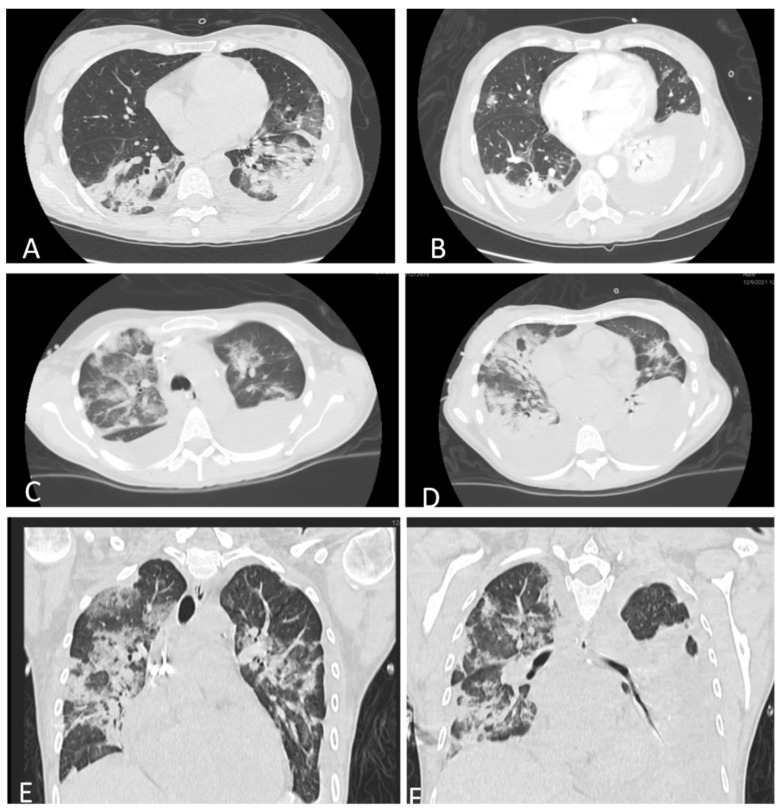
Thoracic CT scan. (A) Native thoracic scan showing bilateral COVID-19 pneumonia, affecting 30% of the lungs, and minimal right pleural effusion. (B) Contrast-enhanced thoracic CT scan revealing medium right pleural effusion and bilateral COVID-19 pneumonia. (C,D) Native thoracic CT scan, transversal view with bilateral pleural effusions and bacterial pneumonia. (E,F) Native thoracic CT scan, sagittal view with large right pleural effusion, inferior right lobe atelectasis, and bacterial pneumonia.
